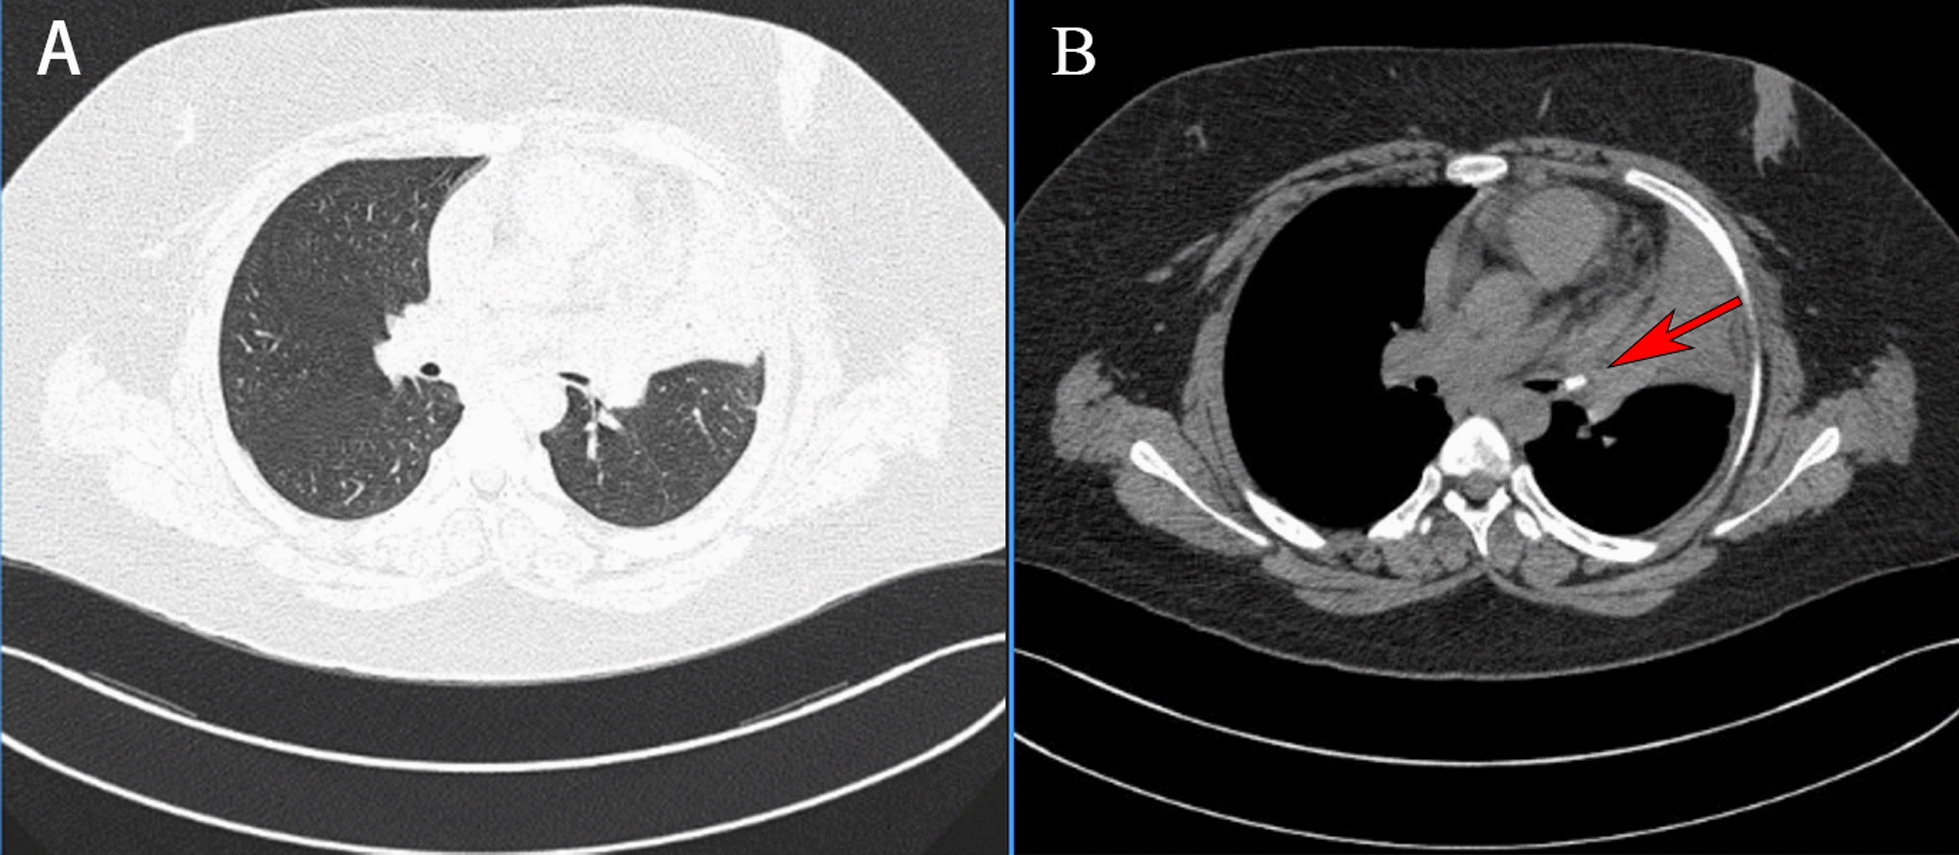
Chest computed tomography images of the child. A, B chest computed tomography showing a high-density shadow (red arrow) in the main bronchial lumen of the left superior lobe with partial atelectasis
